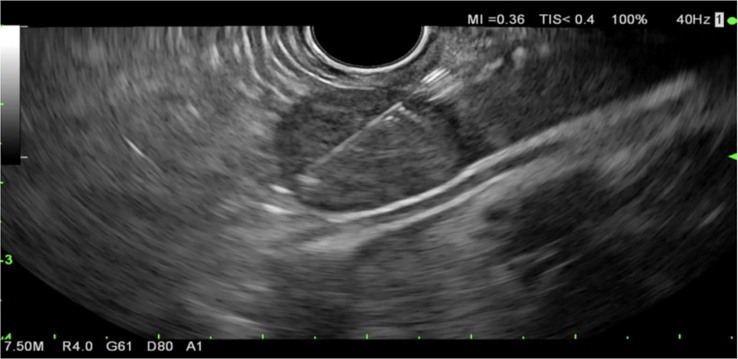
EUS-guided core biopsy of an isoechoic lesion in the left lobe of the liver. Abbreviation: EUS, endoscopic ultrasound.
